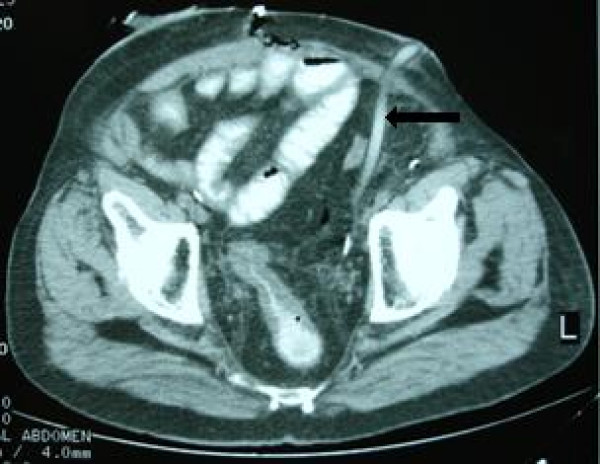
CT scan at case presentation. Computed tomography demonstrates gastrografin into the drain.
